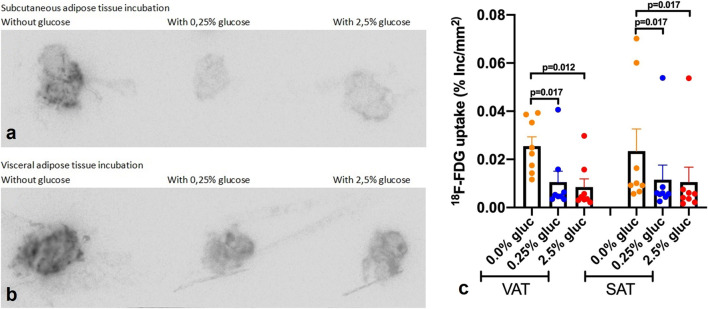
Incubation with glucose decreases [^18^F]FDG uptake in adipose tissue. Autoradiography visualized effect of *ex vivo* incubation of AT with different concentrations of glucose (0 %, 0.25 % and 2.5 %) on [^18^F]FDG uptake in subcutaneous adipose tissue (SAT) (a) and visceral adipose tissue (VAT) (b). Quantification of [^18^F]FDG uptake (in percentage of incubated dose per area in mm^2^ (%Inc./mm^2^)) in VAT and SAT, determined with autoradiography (c).
